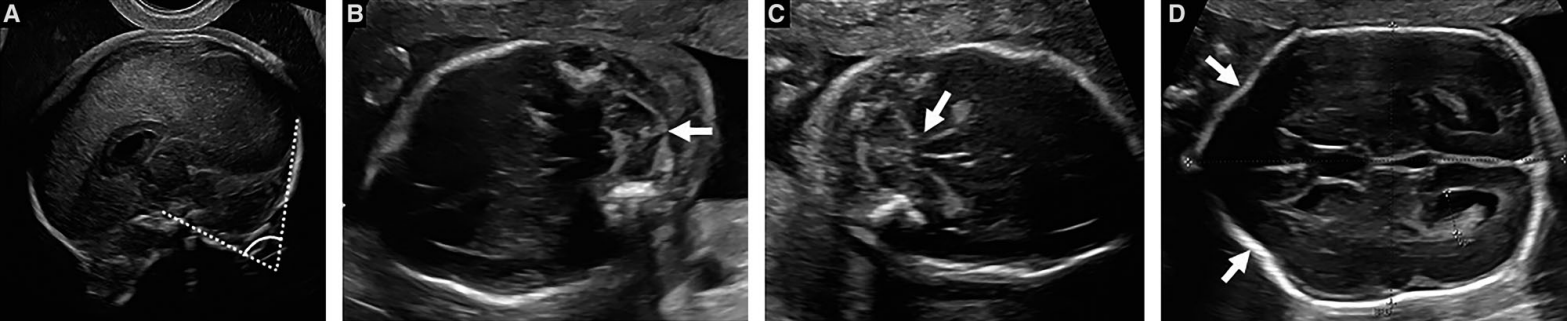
Classic Chiari II malformation findings detected by ultrasound in fetuses with open spina bifida: (A) midsagittal view demonstrating a clivus–occipital bone angle of 69°; (B) transcerebellar view showing a ‘banana’ sign of the cerebellum and an effaced cisterna magna; (C) transcerebellar view showing prominent beaking and elongation of the tectum; (D) transventricular view demonstrating the scalloping of the frontal bones of fetal skull, known as the ‘lemon’ sign. Reprinted with permission of the UZ Leuven, Leuven, Belgium.
